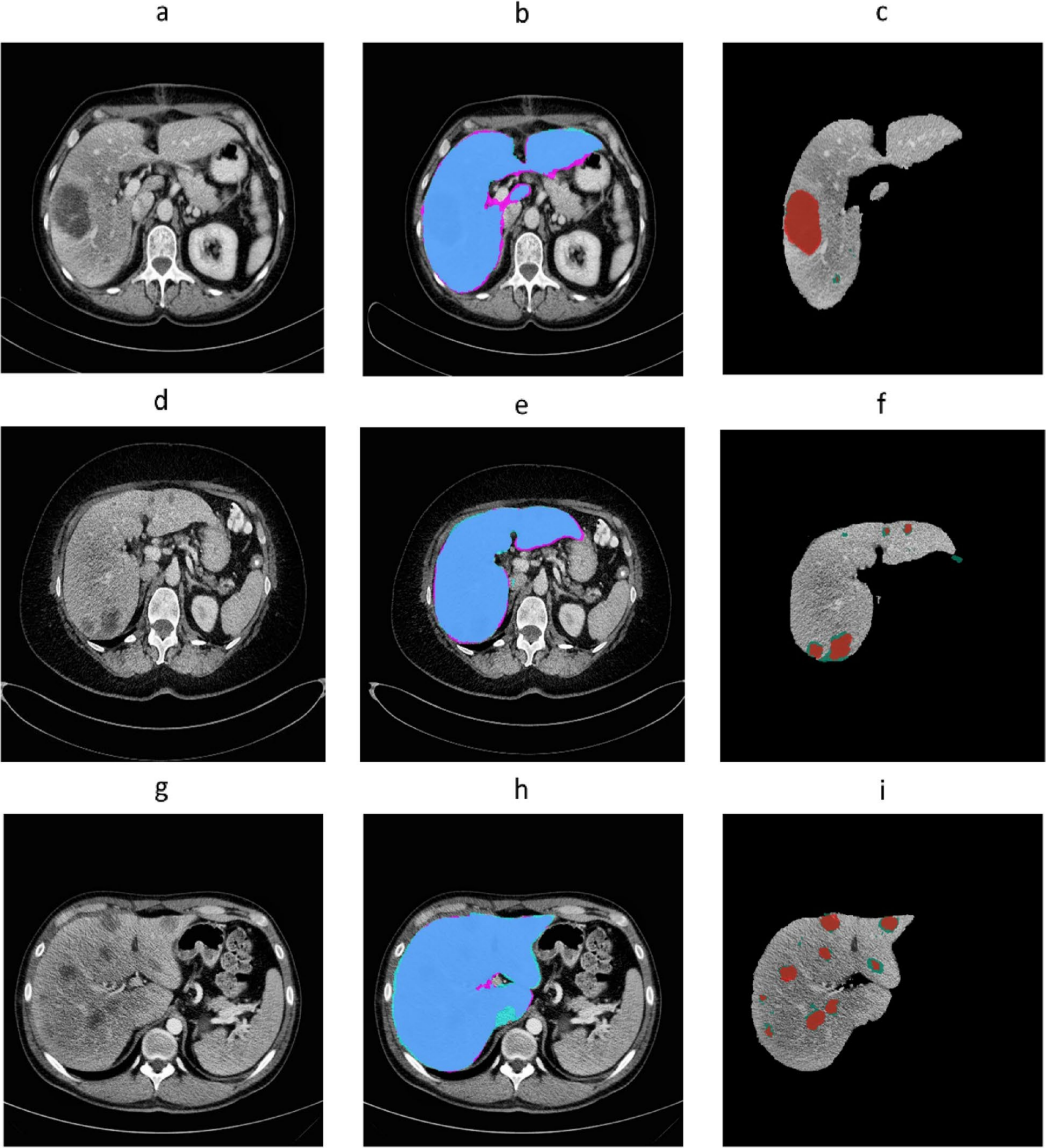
Automatic segmentations of the liver and colorectal liver metastases in three patients of the development cohort. a, d, g Computed tomography scans before automatic segmentation. b, e, h Radiologist’s liver segmentation (pink) and automatic liver segmentation (blue). c, f, i Radiologist’s tumor segmentation (dark green) and automatic tumor segmentation (red)
